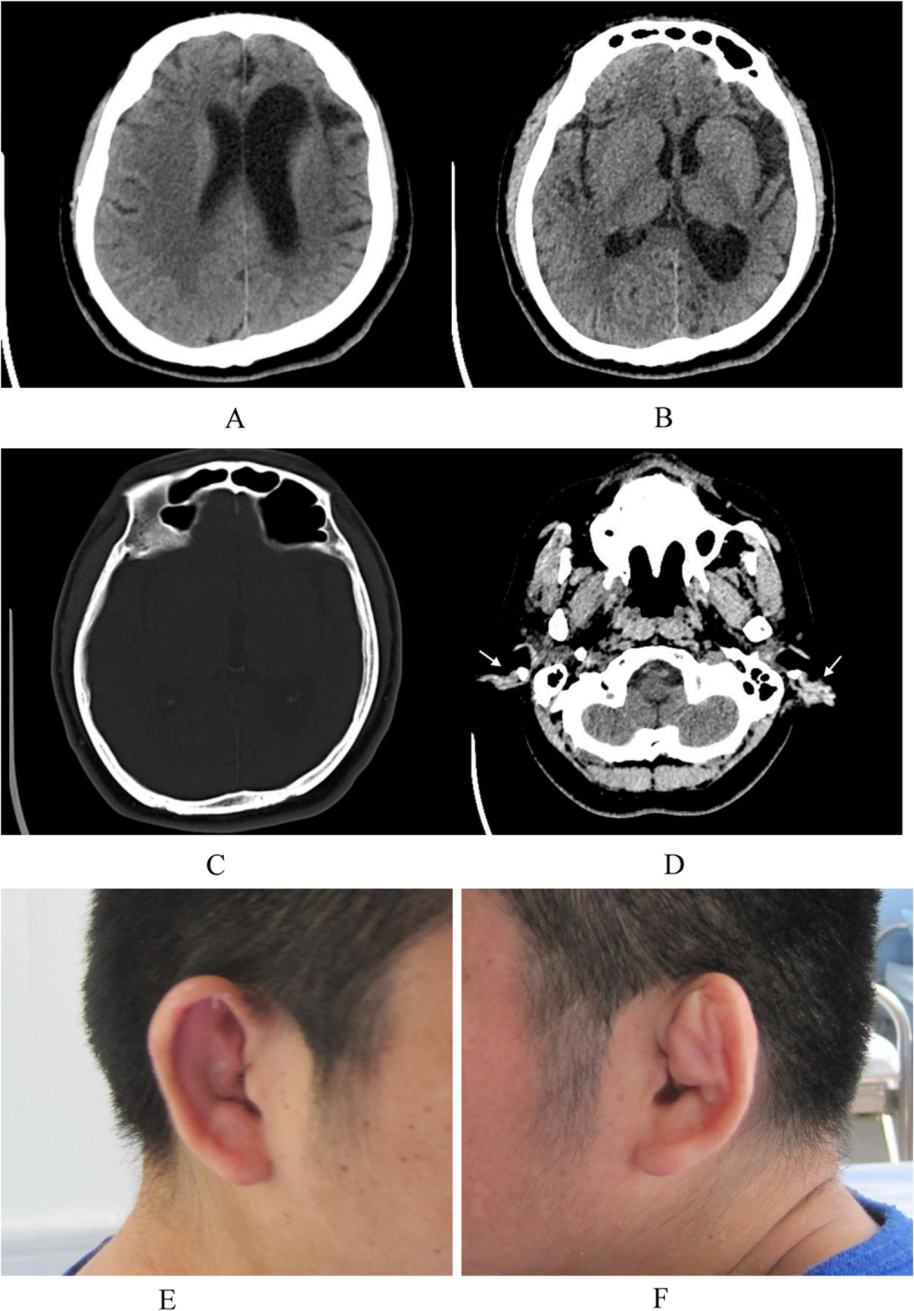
A 30-year-old male patient with DDMs and deformities of external ears (Case3). Axial CT showed left-sided frontal (a) and temporal (b) lobe hemiatrophy, and ipsilateral hyperaeration of the frontal sinus (c), the soft tissue thickening with calcification of bilateral external auricles (d). The corresponding photos of right (e) and left ears (f)
